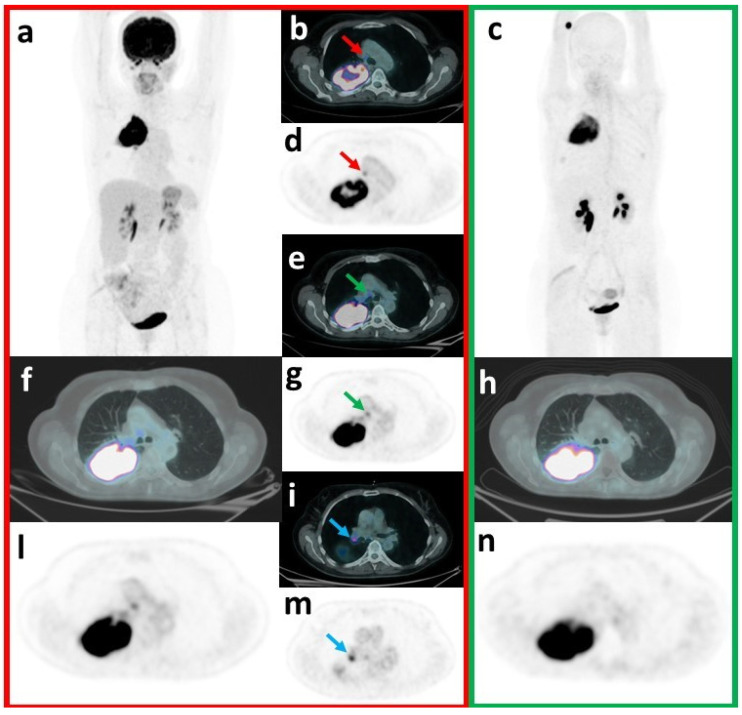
N-FAPI True negative—FDG False positive. A 60 y.o. woman underwent a diagnostic CT to investigate the onset of persistent cough, asthenia, weight loss, fever and haemoptysis. Diagnostic CT revealed the presence of a voluminous lung lesion in the right superior lobe, requiring PET/CT staging. FDG PET/CT detected intense and inhomogeneous uptake (SUVmax = 28.8) in the known lung lesion (a) FDG-MIP-maximum intensity projection; (f) transaxial PET fused with the attenuation correction-CT [ACCT]; (l) FDG transaxial PET). Significant uptake was also observed respectively in the right lower paratracheal (#4; SUVmax = 4.3; (b) red arrow on transaxial PET fused with the ACCT; (d) red arrow on FDG transaxial PET), subcarinal (#7, SUVmax = 4.9; (e) green arrow on transaxial PET fused with the ACCT; (g) green arrow on FDG transaxial PET) and right hilar ln stations (#10, SUVmax = 8.9; (i) blue arrow on transaxial PET fused with the ACCT; (m) blue arrow on FDG transaxial PET), suggestive for LNM (T3N2M0). FAPI PET/CT also confirmed the intense and inhomogeneous uptake in the lung lesion (SUVmax = 22.5; (c) FDG-MIP-maximum intensity projection; (h) transaxial PET fused with the ACCT; (n) FDG transaxial PET), but did not detect any significant lymph nodal uptake (T3N0M0). Endobronchial ultrasound-guided biopsies resulted positive in the lung for squamous cell carcinoma but negative for metastasis in the right lower paratracheal, subcarinal and right hilar lymph nodes. Surgery confirmed biopsies results: a poorly differentiated squamous cell carcinoma, with pleural, bronchial and vascular invasion, was diagnosed without lymph nodal involvement (pT3N0Mx). Subsequently, the patient started chemotherapy.
